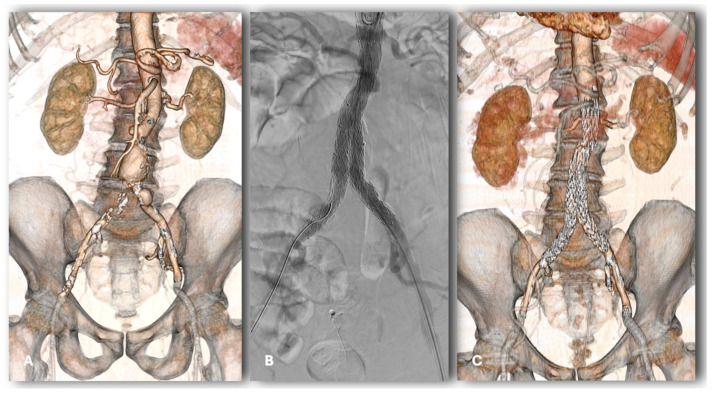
Pre-operative CT-angiogram of a patient with AAA and chronic total occlusion of the right common iliac artery (A); final intraoperative angiography and post-operative CT-angiogram demonstrating effective Incraft implantation with sac exclusion and complete right common iliac artery recanalization (B,C).
